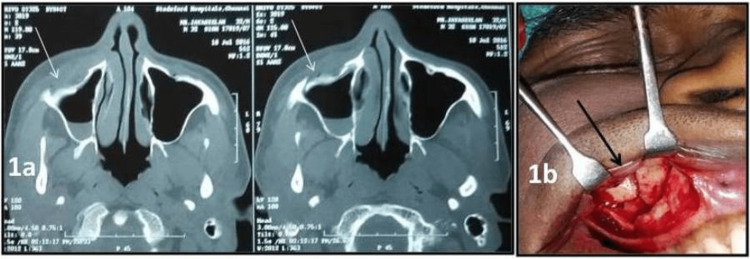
1a: comminuted fracture of the anterior wall of the maxillary sinus as evident in the CT scan axial section (preoperative); 1b: clinical picture of fractured segments
